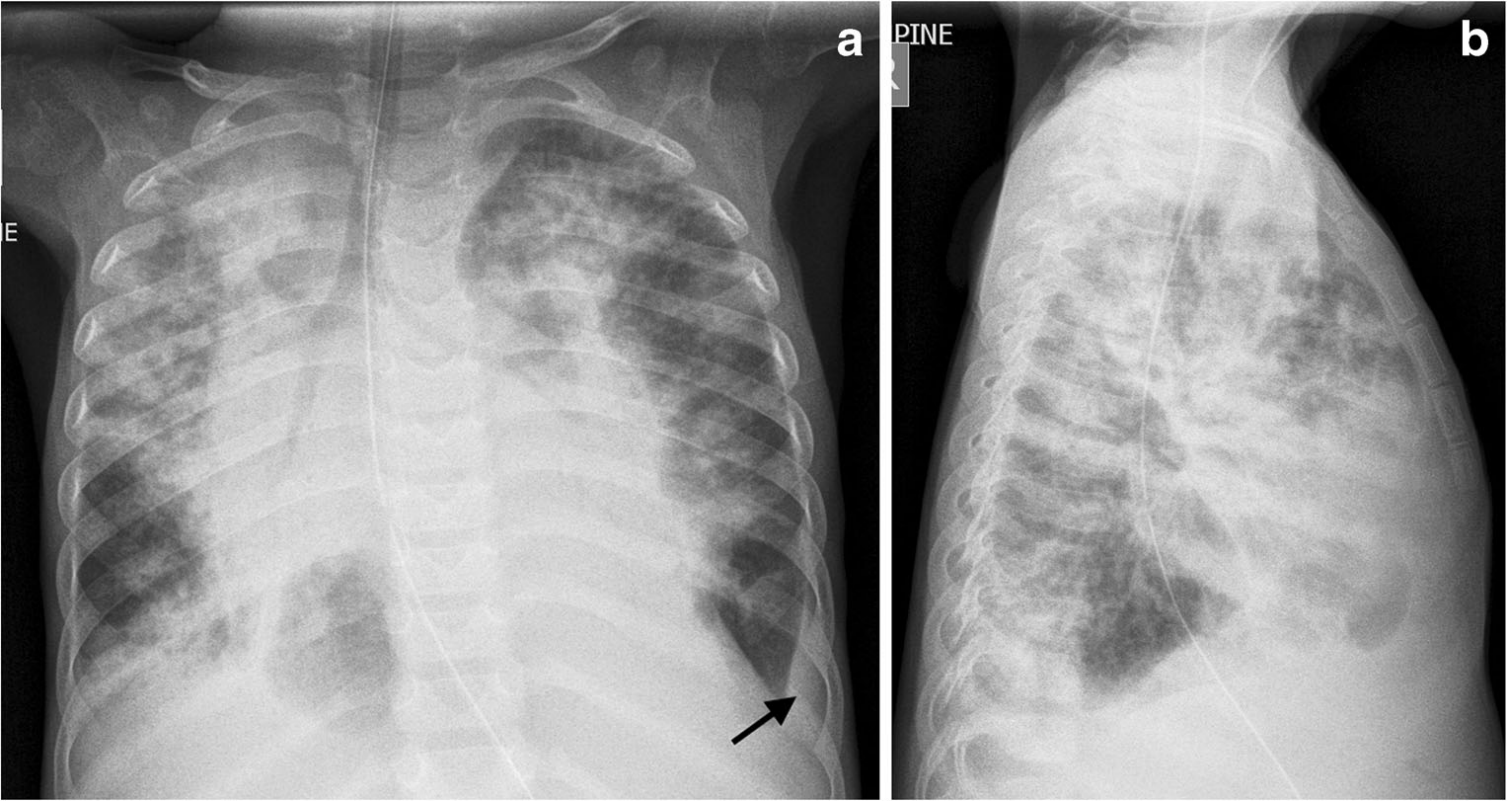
Imaging in a 6-year-old boy presenting with cough, dyspnoea, hepatosplenomegaly and oedema at a Médecins Sans Frontières site in the Democratic Republic of Congo. a, b Anteroposterior (a) and lateral (b) chest radiographs. The report read: “CXR shows widespread consolidation — there are many causes for multifocal pneumonia like this. TB is also possible as there is a small left basal pleural effusion (*black arrow*) and probable mediastinal adenopathy”. A round lucency in the right lower zone was reported as aerated lung and not a cavity
